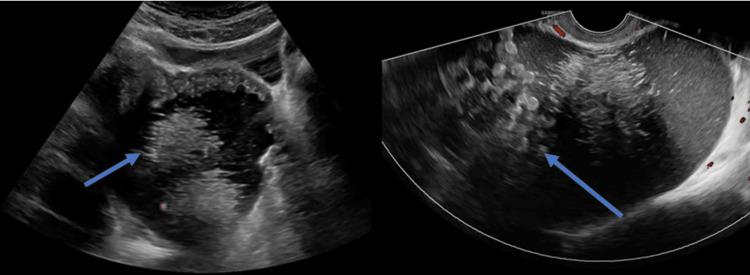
Pelvic ultrasonography showing right-sided ovarian cystic cavity with multiple small hyperechoic dots without internal flow (blue arrows).
